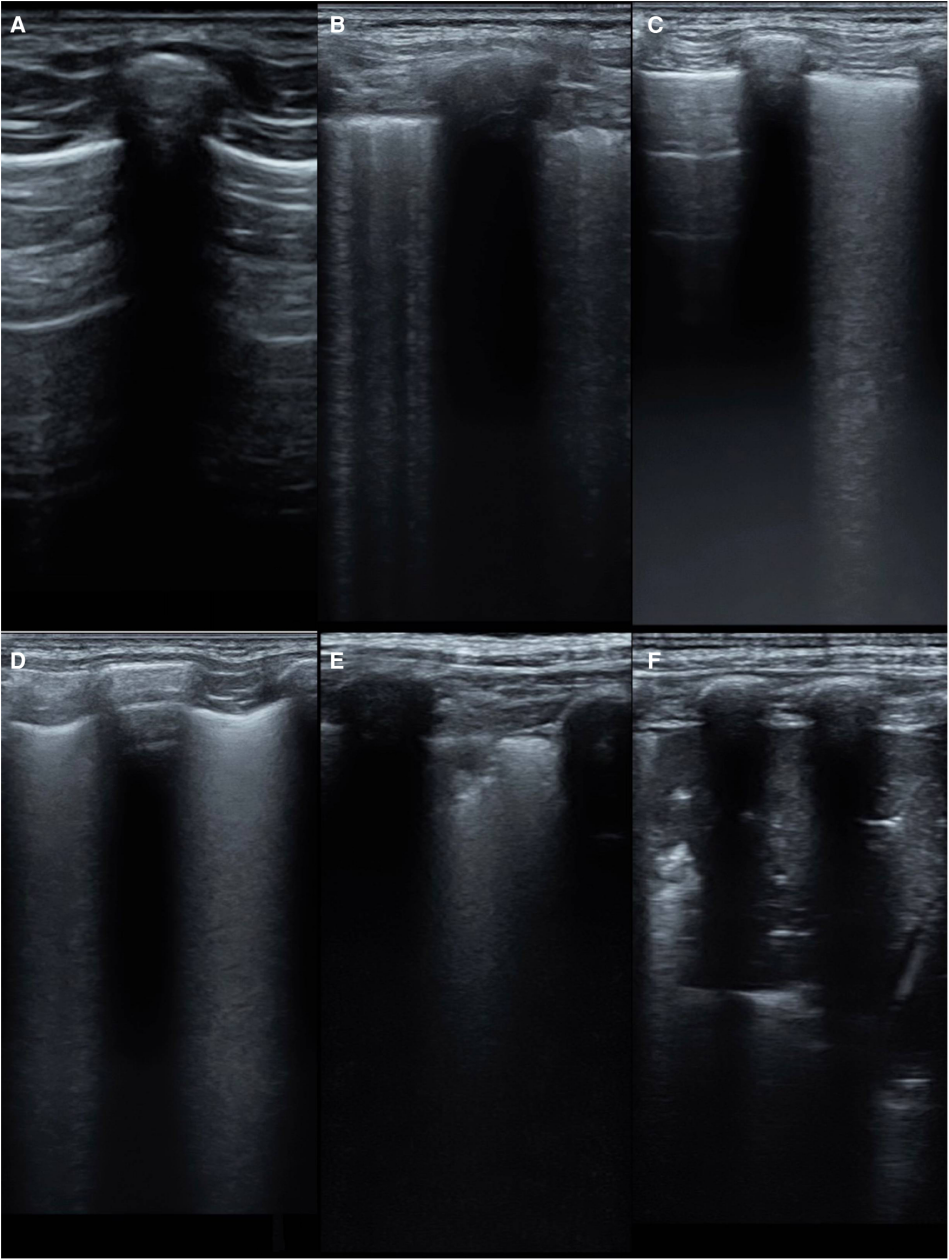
The scoring criteria for the modified lung ultrasound images. (A) A-line is dominant, <3 non-fused B-lines(0 point); (B) multiple non-fused B-lines(1 point); (C) dense, partially fused B-lines(2 points); (D) completely fused B-lines(3 points) (E) abnormal pleural line with a small range (depth <1 cm) of subpleural lung consolidation(4 points); (F) abnormal pleural line with large-area (depth >1 cm) lung consolidation(5 points).
